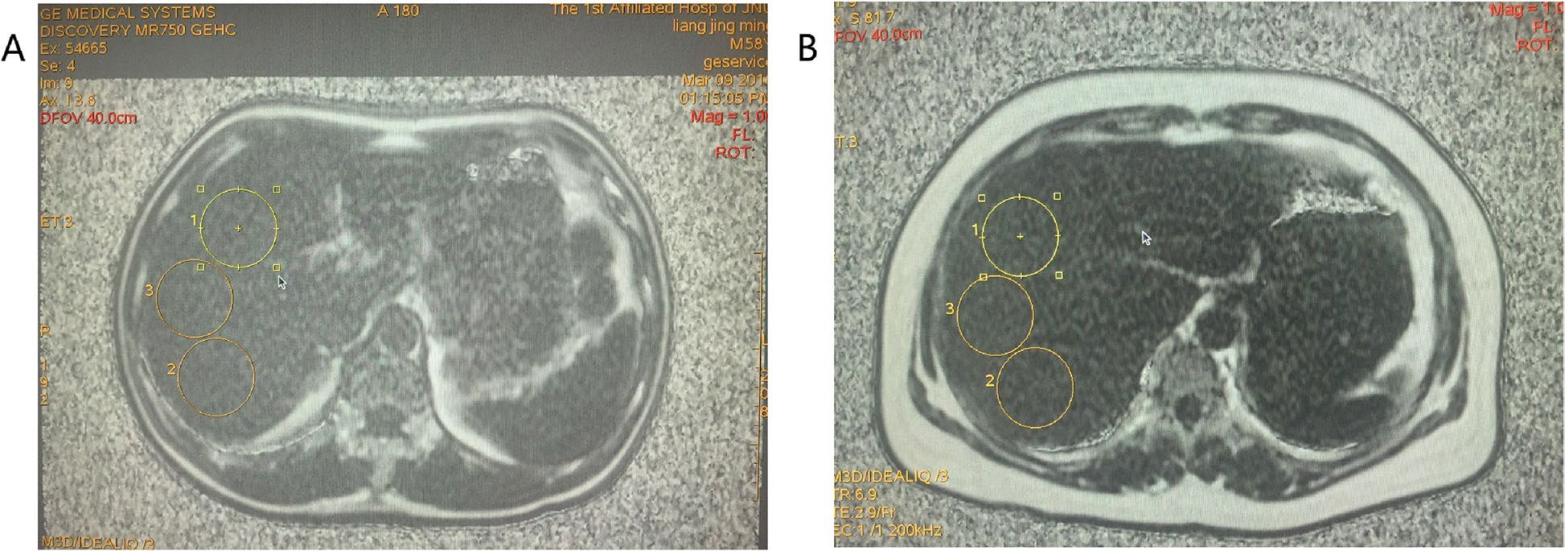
Location of the region of interest (ROI) in MRI IDEAL-IQ Sequence. A In-phase image of the region of interest. B Out-of-phase image of the region of interest
